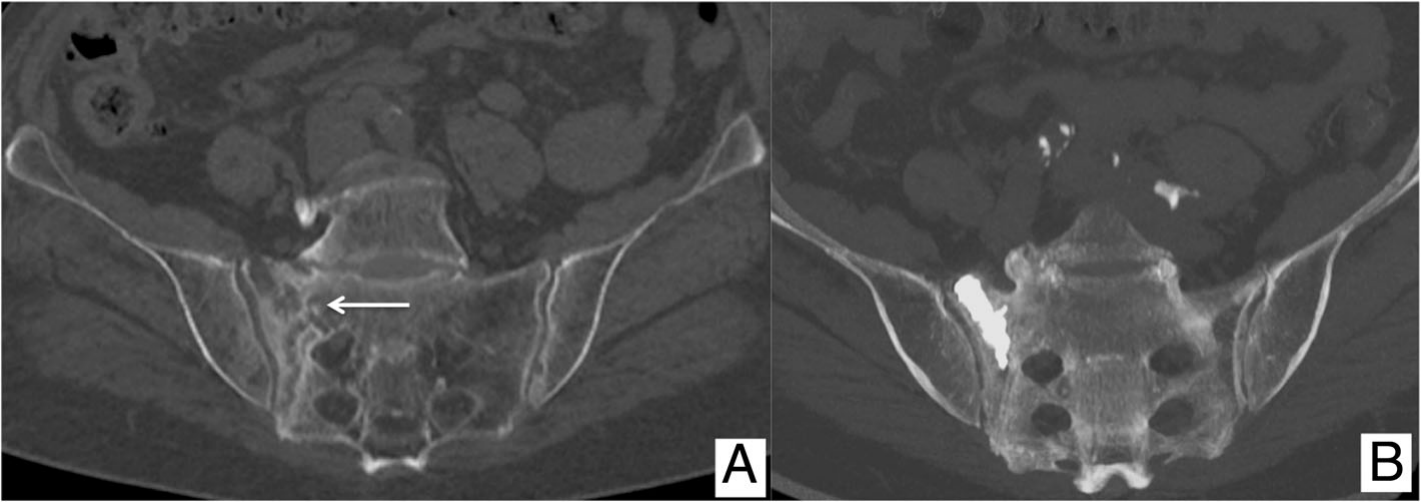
Eighty-four-year-old female patient with breast cancer, presenting with (a) a painful insufficiency fracture of the right sacral wing (arrow). b Coronal MIP CT image demonstrating the result of the sacroplasty
